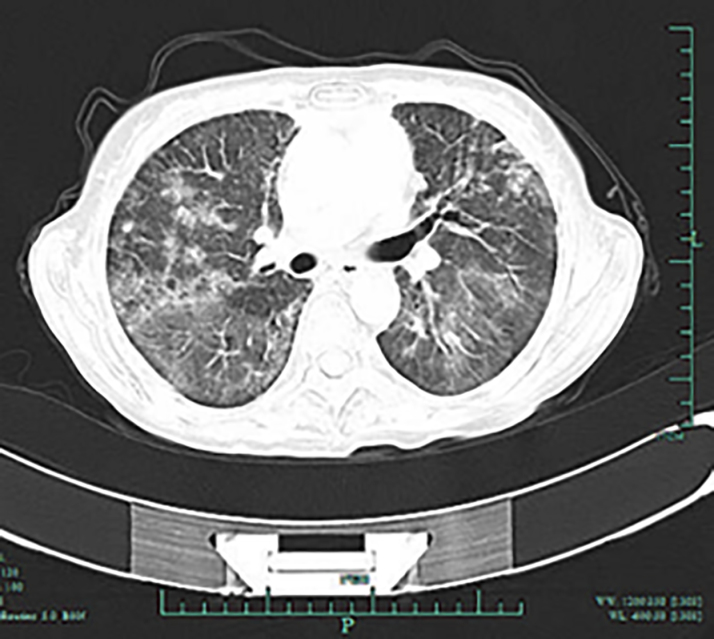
Chest CT image acquired on September 15, 2016. The scan reveals bilateral pulmonary infiltrates, predominantly involving the lower lobes, consistent with recurrent aspiration pneumonia or opportunistic infection. Microbiological workup later identified *Pseudomonas aeruginosa* as the causative pathogen. This finding reflects the profound susceptibility to respiratory infections in patients with Good syndrome. CT = computed tomography.
